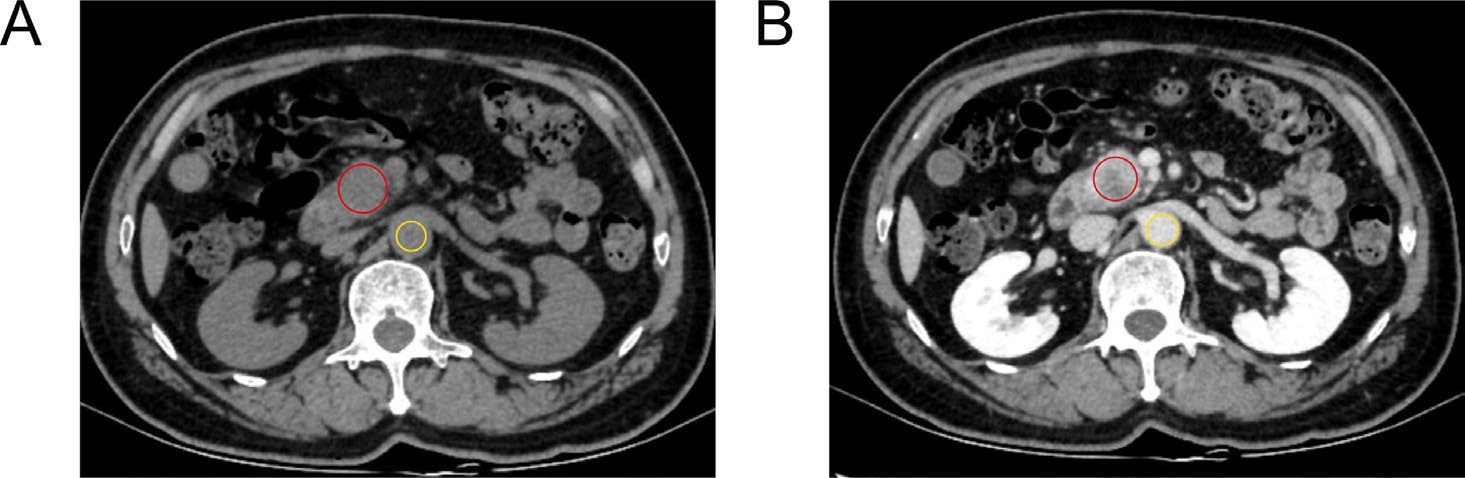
In the non‐enhanced axial scans (A) and the equilibrium axial scans (B) of CT image, ROI selection is performed (the red ellipse represents the ROI of the PDAC lesion, and the yellow ellipse represents the ROI of the abdominal aorta). (A) In the non‐enhanced image, attenuation values of the PDAC lesion and the abdominal aorta are 37 HU and 47 HU, respectively. (B) In the equilibrium phase image, attenuation values of the PDAC lesion and the abdominal aorta are 92 HU and 172 HU, respectively. This patient's HCT is 0.422, ΔHU^tumor^ is 55, ΔHU^aorta^ is 125. According to Formula (1), the patient's fECV is calculated to be 25.43.
